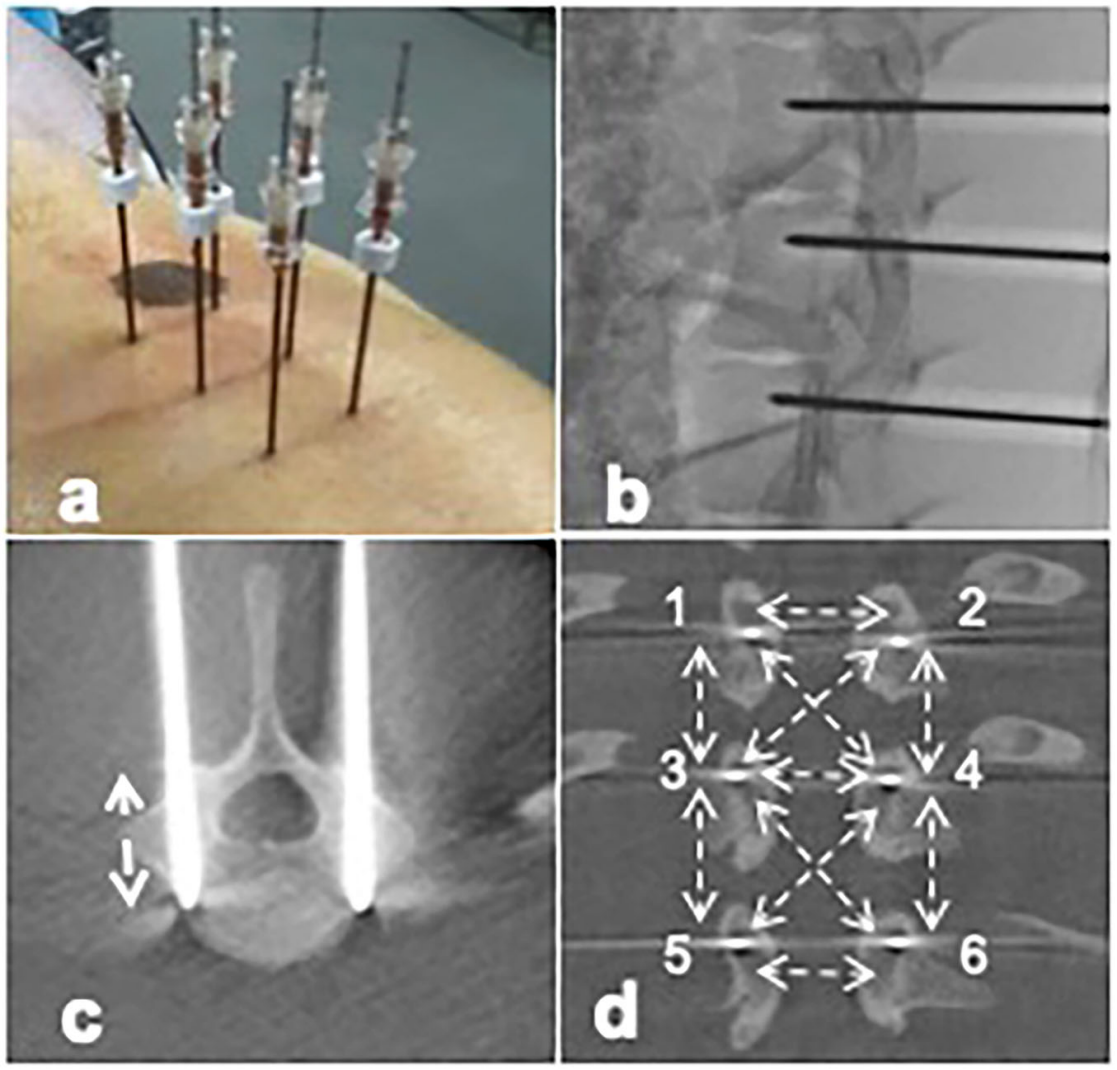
Percutaneous insertion of 6 electrodes (a) was performed under fluoroscopic guidance (b) using a bilateral transpedicular approach at three consecutive levels (T11, T12, and L1). The tip of each electrode was advanced in order to expose the active part (20 mm) at the level of the central canal and the spinal cord (c). Distances between electrodes were measured with cone-beam computed tomography (d) in order to deliver electroporation impacts using a voltage-to-distance ratio of 1,000 V/cm between all electrodes at adjacent levels, with a maximum voltage of 3,000 V
